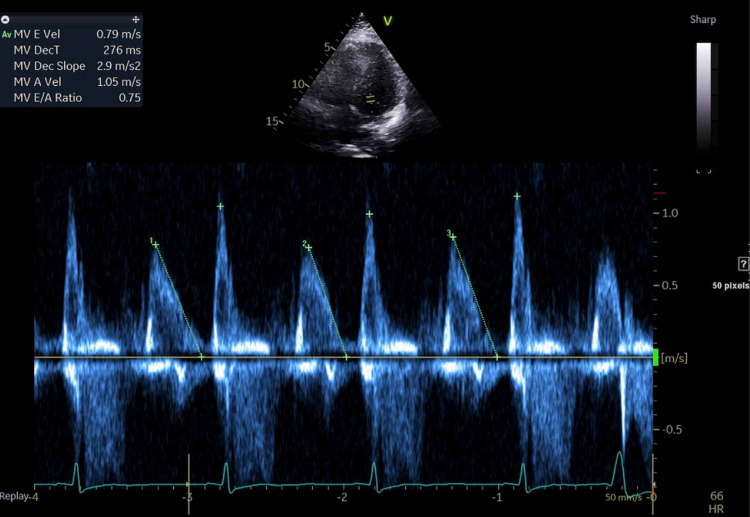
Apical short-axis view, TTE: showing a mitral valve E/A ratio of 0.75 with a deceleration time of 276 ms, consistent with a grade 1 diastolic dysfunction TTE: transthoracic echocardiogram
